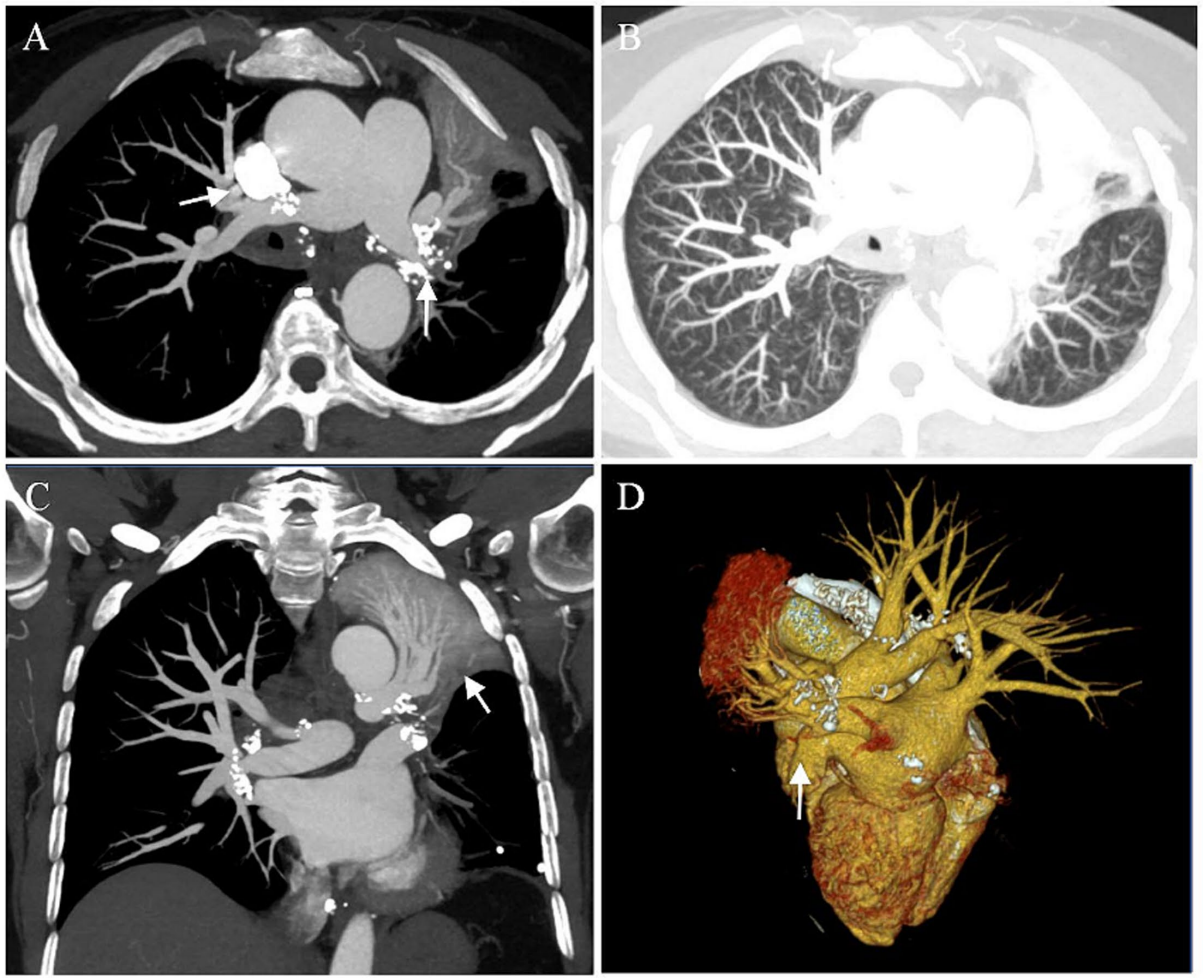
Preoperative images. (A–C) Chest CT angiography showed atelectasis of the left upper lung, multiple calcifications of the hilum, occlusion of the left lower pulmonary artery and vein (arrows). (D) 3D reconstruction demonstrated arteriovenous occlusion of the left lower lobe (arrow).
